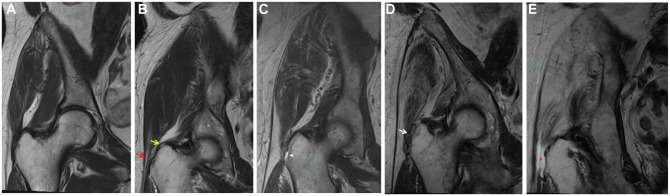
Coronal T2-weighted magnetic resonance images of a right hip
